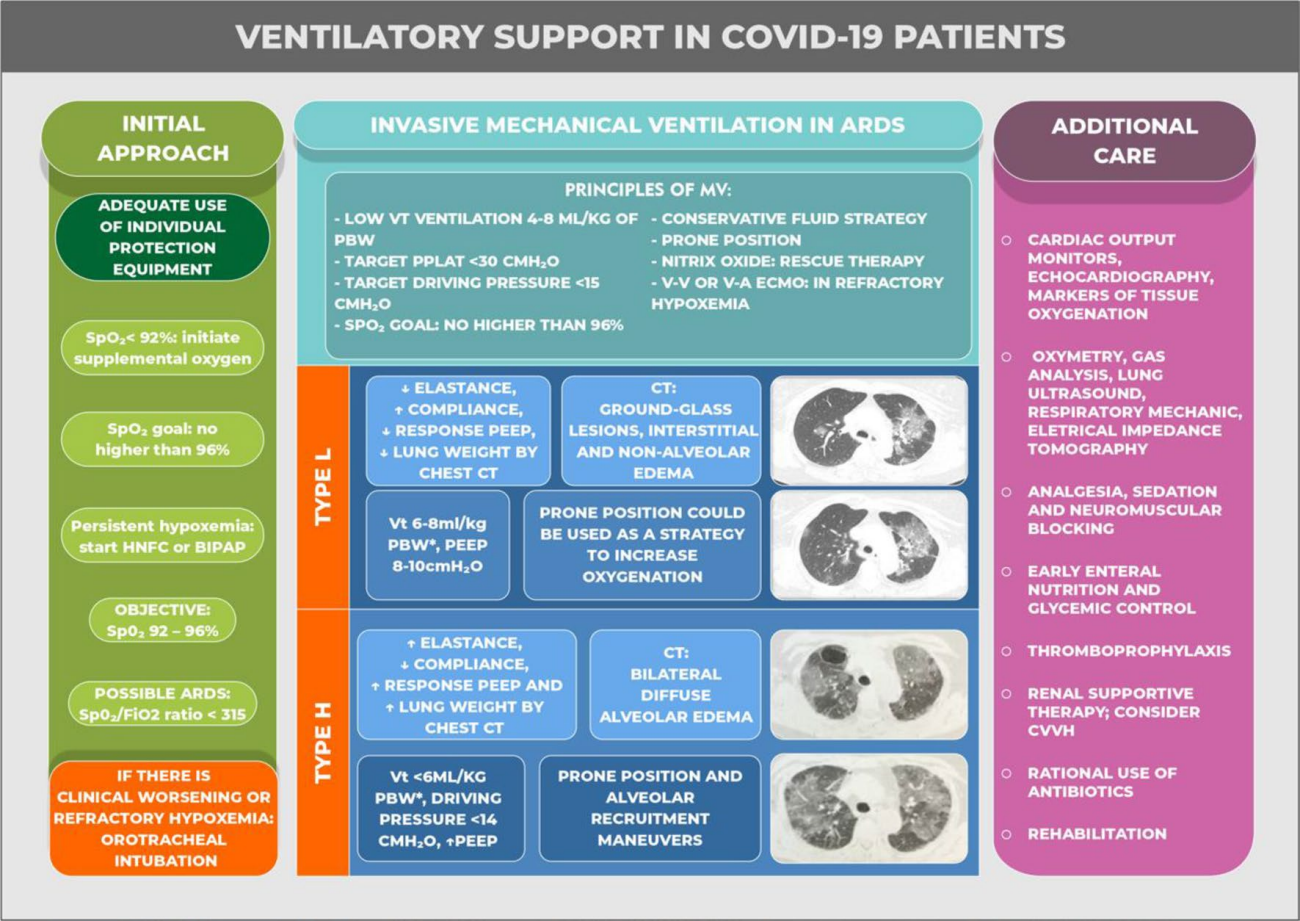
Ventilatory support in COVID-19 patients. *PBW* predicted body weight, *ARDS* acute respiratory distress syndrome, *BNP* brain natriuretic peptide, *CPAP* continuous positive airways pressure, *CT* computerized tomography, *CVVH* continuous venovenous hemofiltration, *CRP* C-reactive protein, *ECMO* extracorporeal membrane oxygenation, *LDH* lactic dehydrogenase, *PEEP* positive end-expiratory pressure, *PPlat* plateau pressure, *HNFC* high-flow nasal cannulas, *NIV* non-invasive ventilation, *PEEP* positive end-expiratory pressure, *SpO2* peripheral O2 saturation, *PaO2* partial pressure of oxygen, *Vt* tidal volume, *V-V* venovenous, *V-A* venous-arterial
